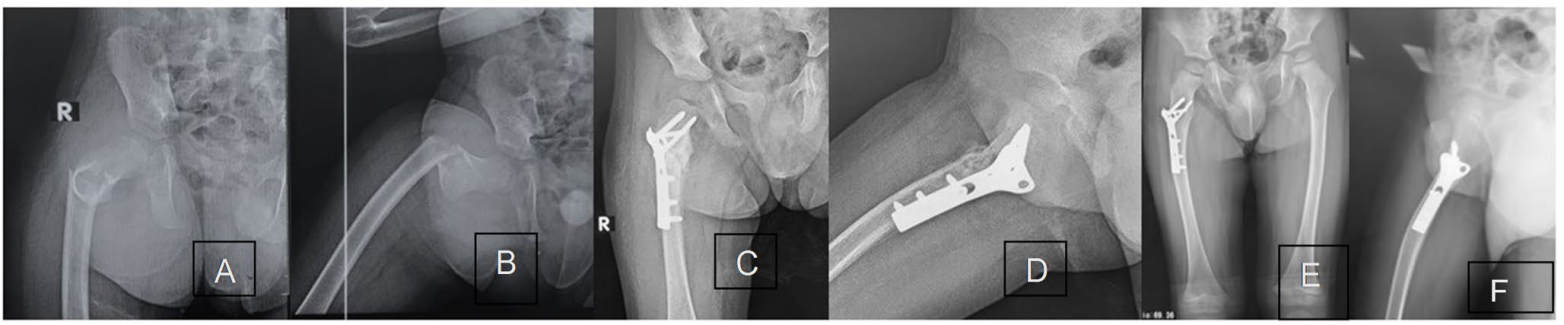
A and B show X-ray anteroposterior and a lateral view of the right hip joint showing a Type V proximal femoral fracture. C and D show Follow-up rays 4 weeks postoperatively, showing the reduction and fixation of the fracture with callus formation at the fracture site. E and F Follow-up X-ray 20 months postoperatively before implant removal, showing the maintained hip radiographic parameters with the normal growth of the proximal femur as compared to the contralateral side
